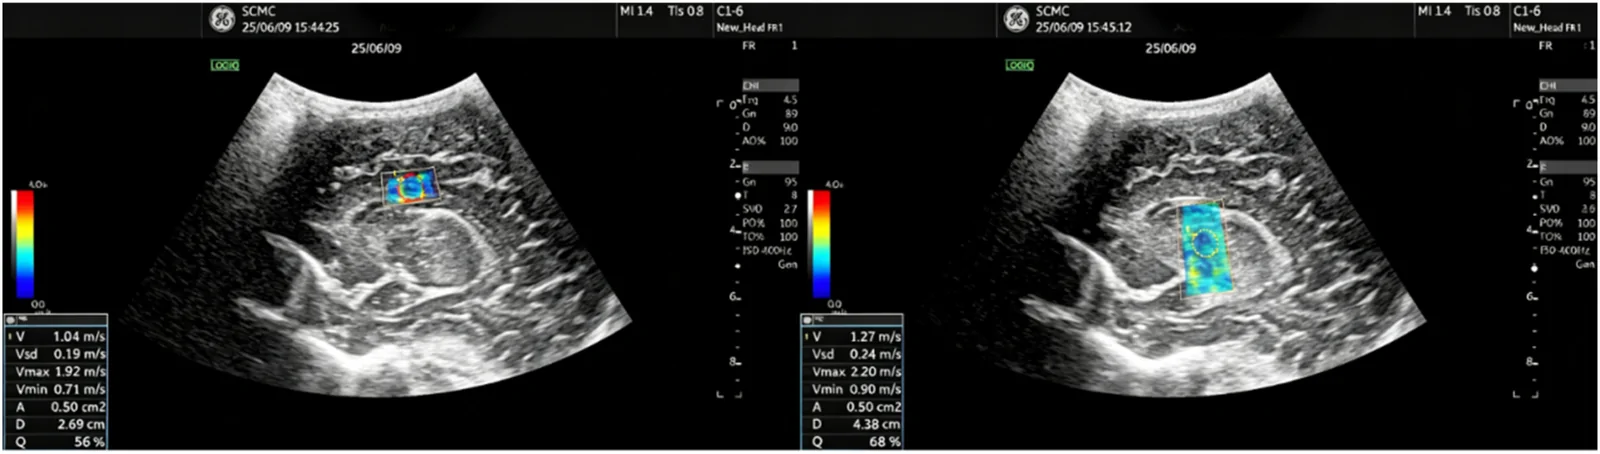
B-mode cranial ultrasound images with shear wave elastography measurements.
